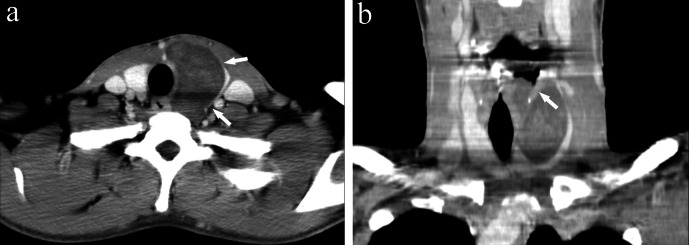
Contrast-enhanced (CT) images. (a) CT demonstrates a solid tumour showing a
